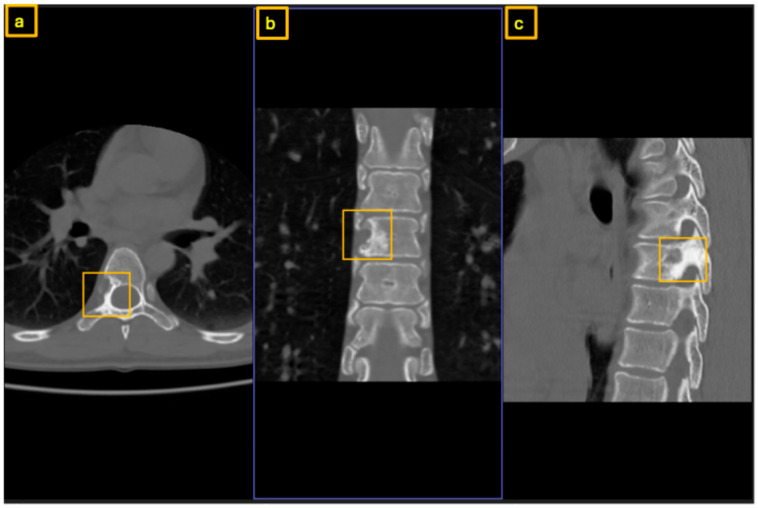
Postoperative CT scan at 3-month follow-up showing recurrence: (a) axial view, (b) coronal view, and (c) sagittal view. The yellow square highlights the recurrent nidus, now larger and infiltrating the right side of the T7 vertebral body.
